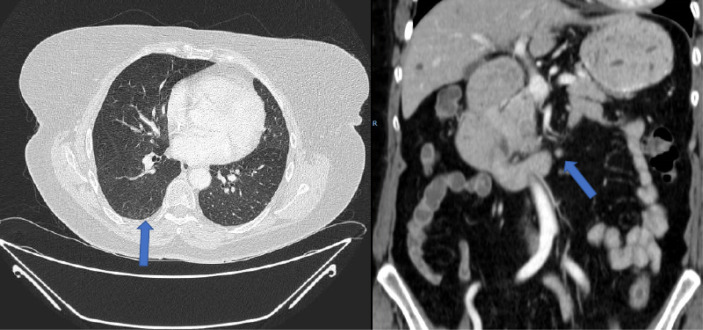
CT after 3 cycles of TAS-102. Complete response of pulmonary metastasis and retroperitoneal lymph node involvement was observed (blue arrows)
